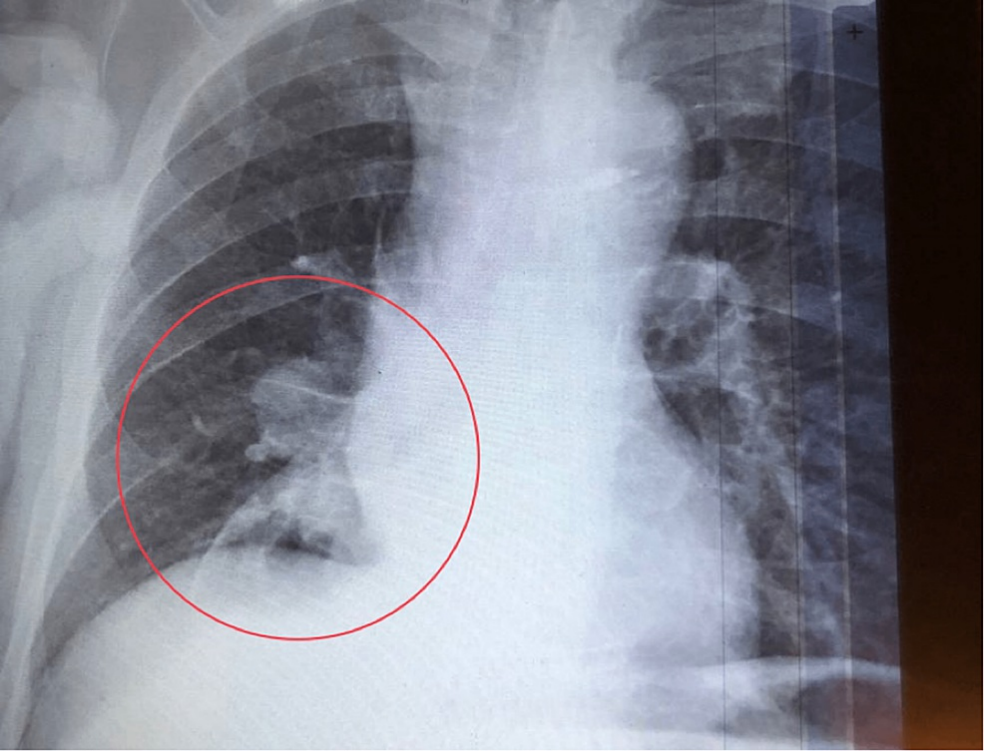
Plain chest radiograph showing right lower lobe lung lesion.
